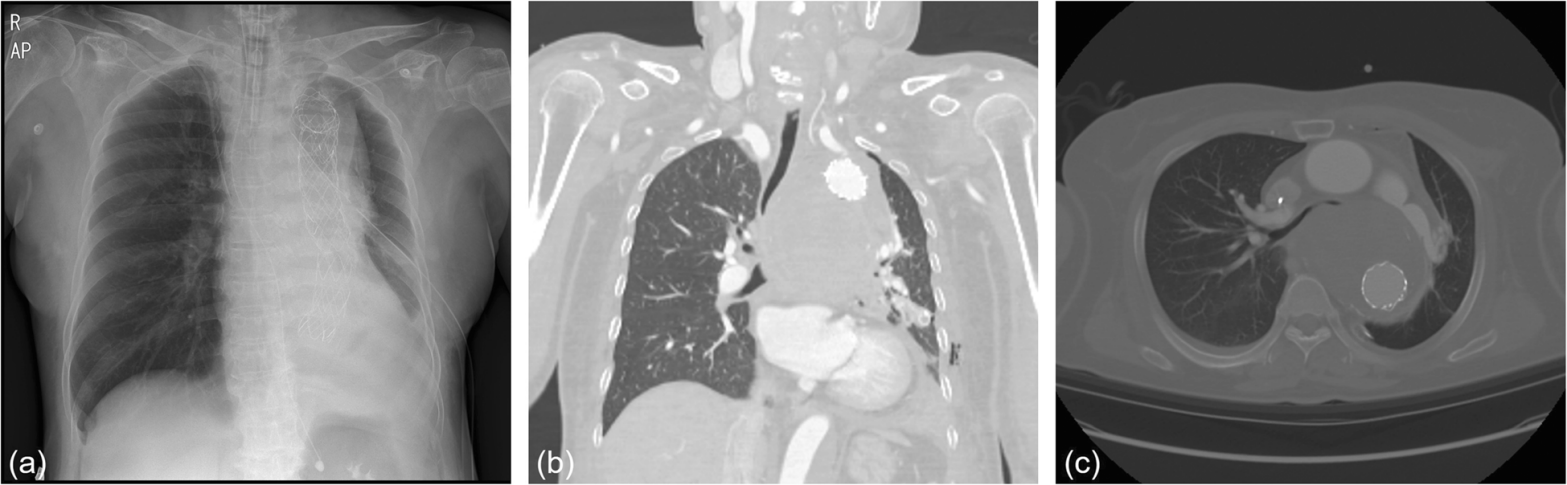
(a) Chest radiography and (b, c) computed tomography of the chest after thoracic endovascular aortic repair (TEVAR, proximal 34 × 30 × 110 and distal 28 × 110). The hematoma of the aneurysm still compressing the lower trachea and both main bronchi
